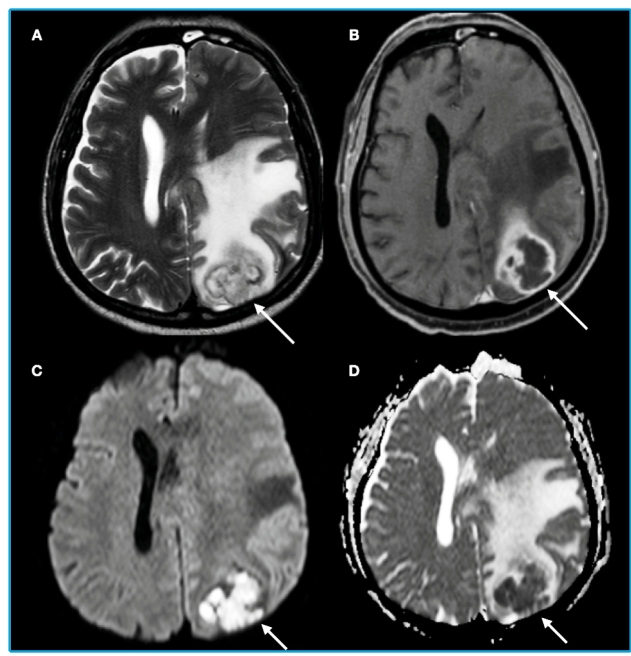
T2 weighted image (A), T1 weighted image after contrast administration (B), diffusion-weighted imaging (DWI) (C) and apparent diffusion coefficient (ADC) map (D), showing expansible lesion in the left parietal lobe, with peripheral ring enhancement (B, arrow), restricted diffusion, characterized by hyperintensity on DWI (arrow, C) and hypointensity on ADC map (D, arrow) and perilesional edema (A).
